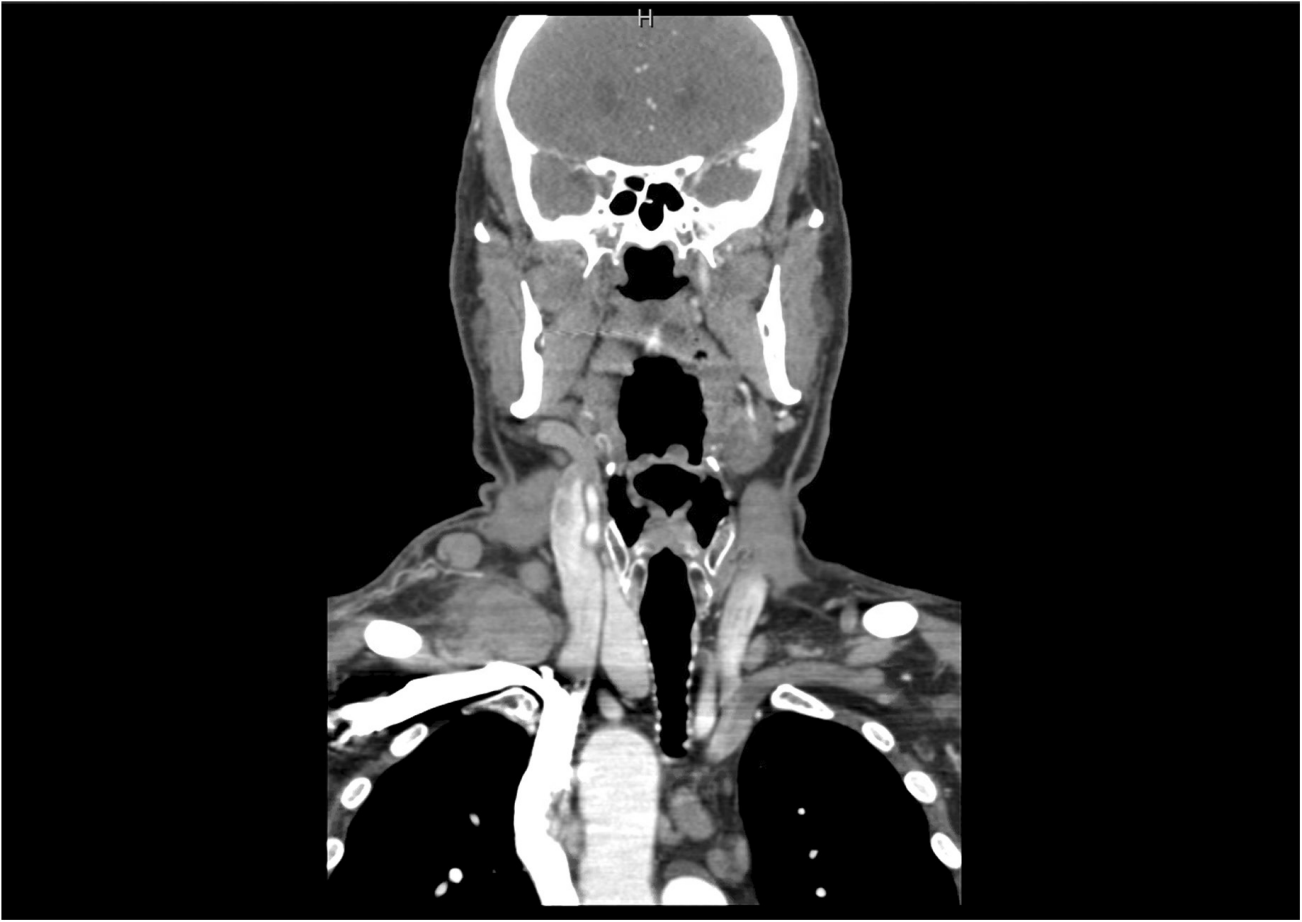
CT soft tissue neck with contrast (transverse view) highlighting large right sided supraclavicular lymphadenopathy.
